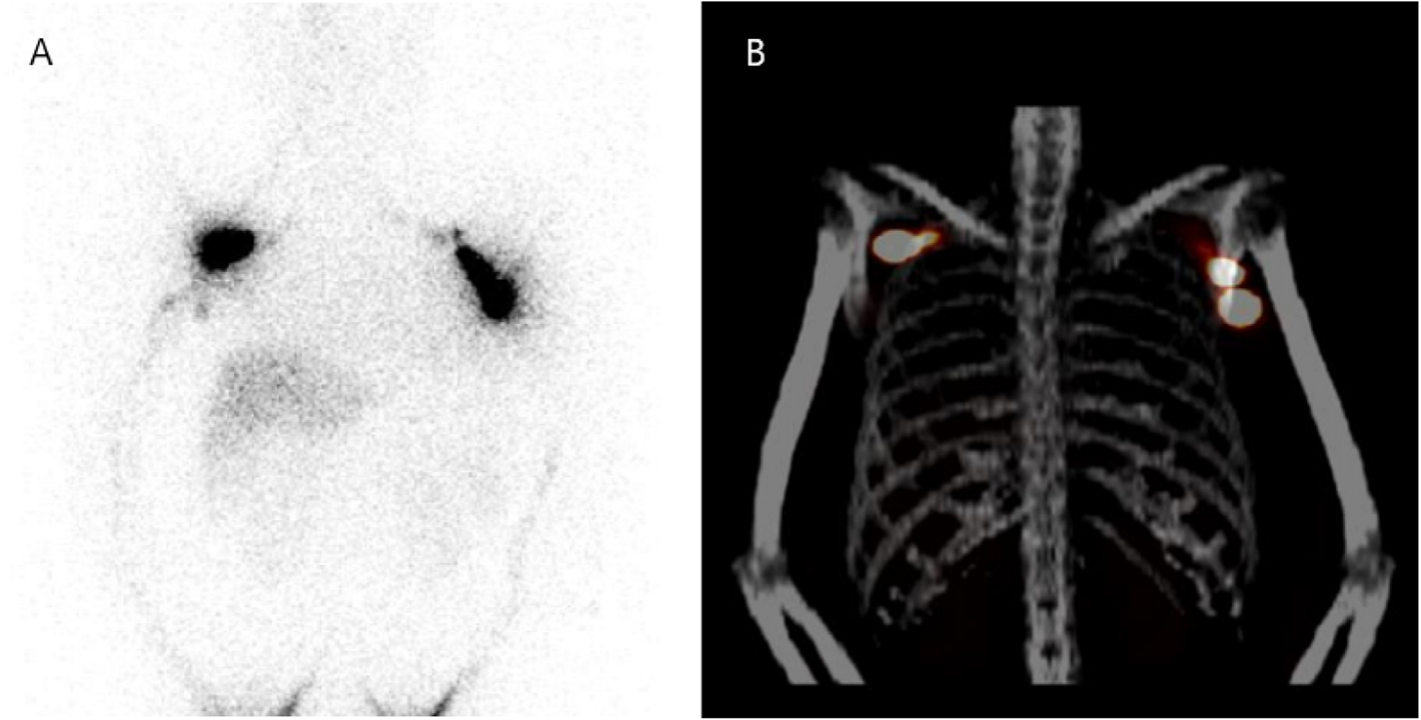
Axillary lymph nodes were visualized with planar scintigraphy (a) and SPECT/CT (b) imaging
